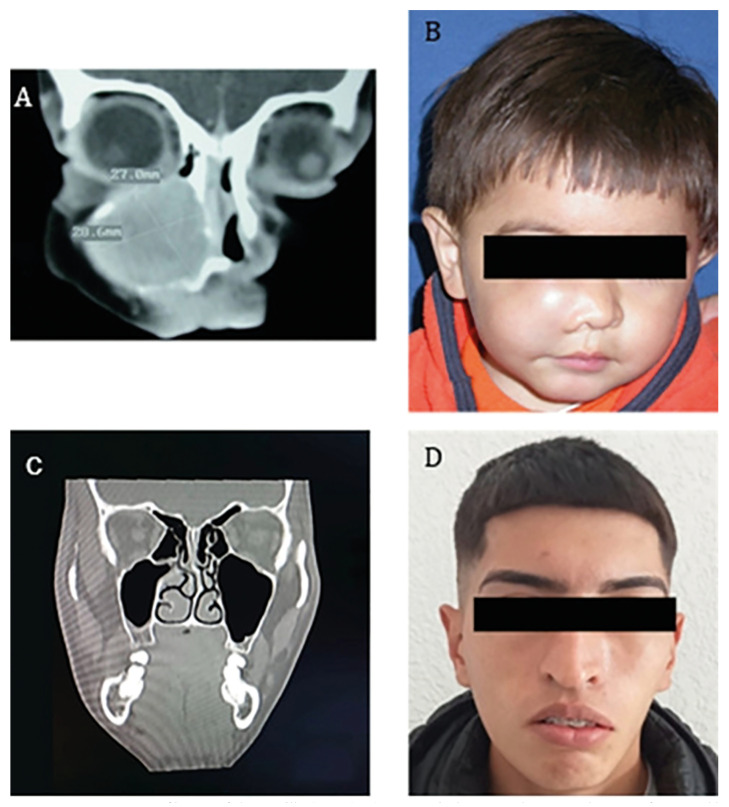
Intraosseous myofibroma of the maxilla (case 2). A) Pre-surgical computed tomography scan of 1-year-old male patient. Note the hypodensity of the lesion and cortical expansion; B) Pre-surgical facial photograph (increase volume corresponding to three months of evolution); C) Computed tomography scan after 17 years of surgical excision. D) Facial photograph after 17 years of treatment.
